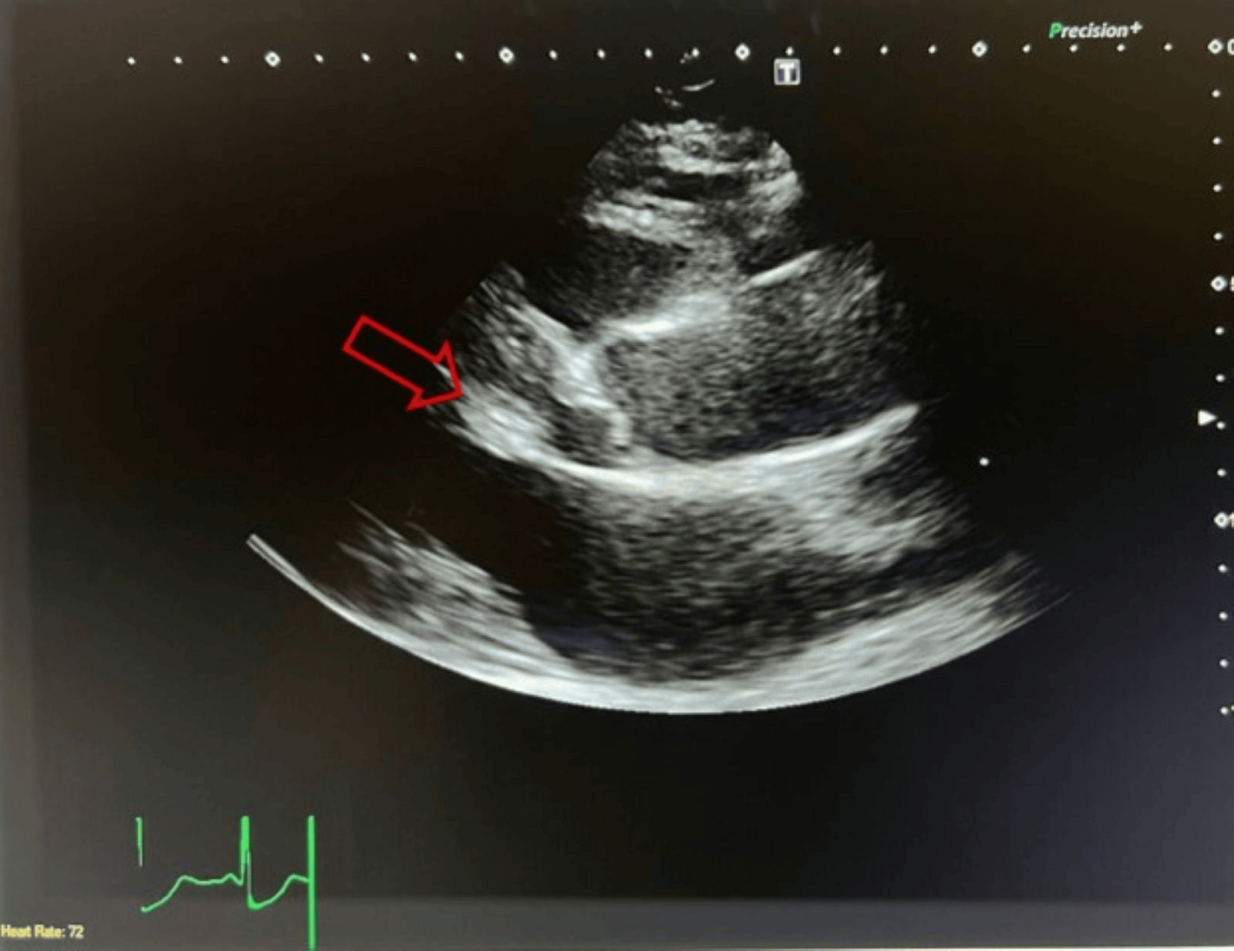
Transthoracic echocardiogram in a parasternal view showed systolic anterior motion of the anterior leaflet of the mitral valve (red arrow).
